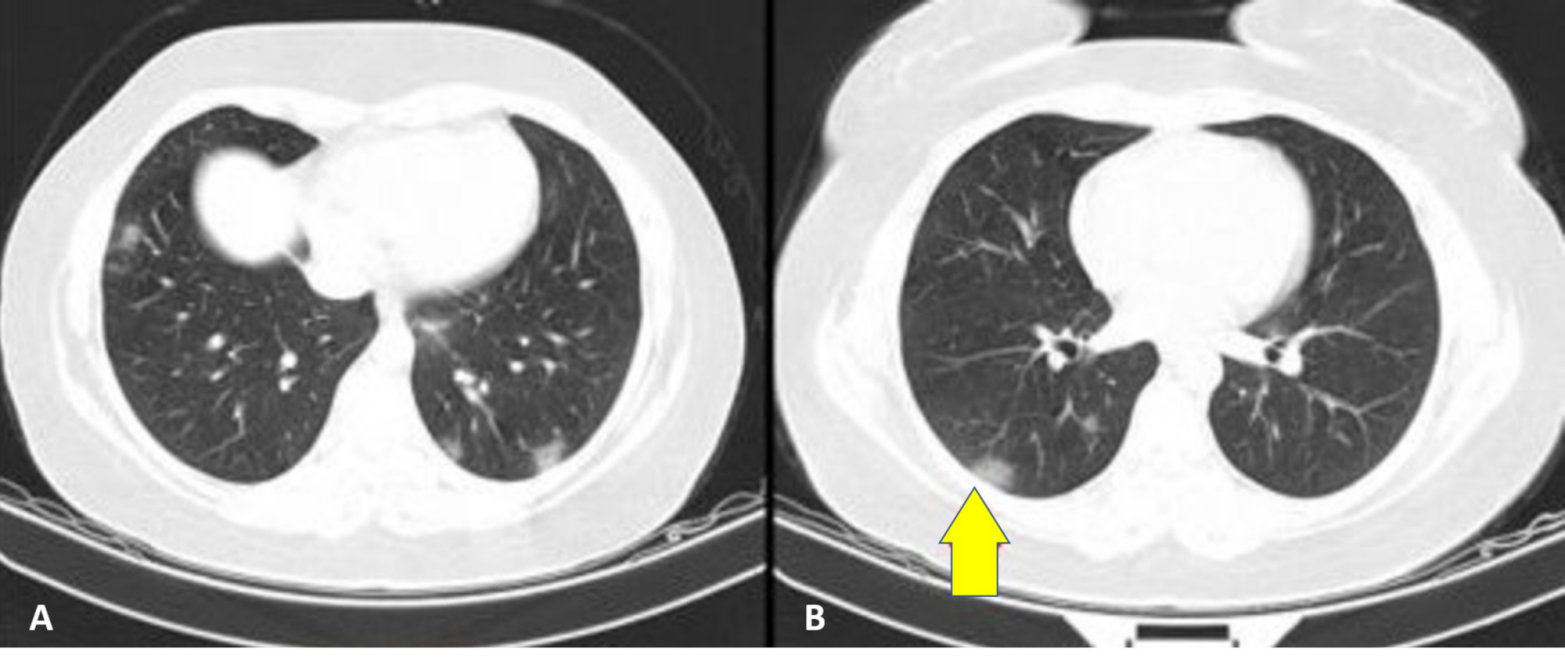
A lung computed tomography scan showing small diffuse patchy consolidation (A) and bilateral ground-glass opacities (B), characteristic of COVID-19 infection
